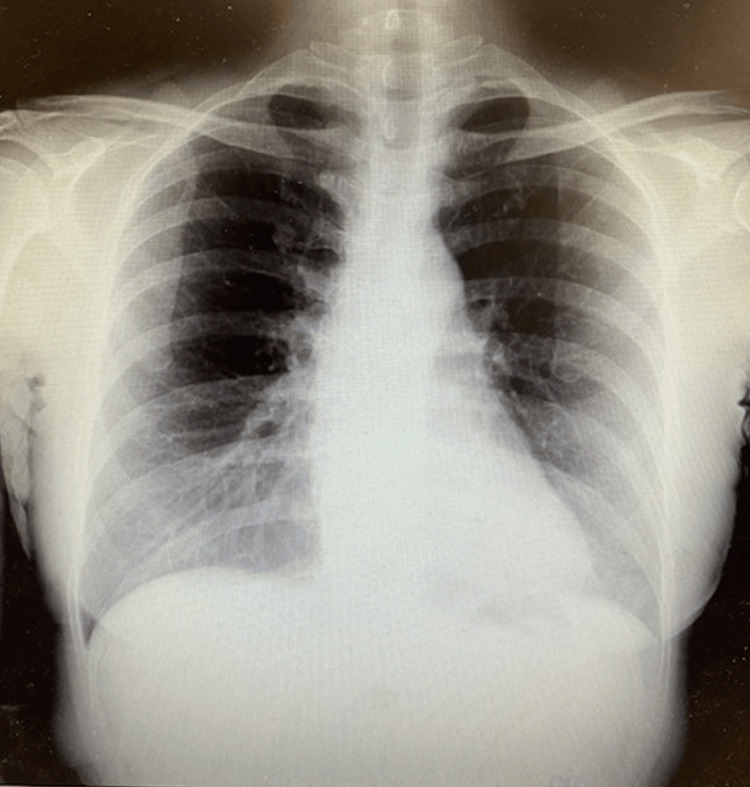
Chest X-ray Chest radiography showed no abnormal shadow.
